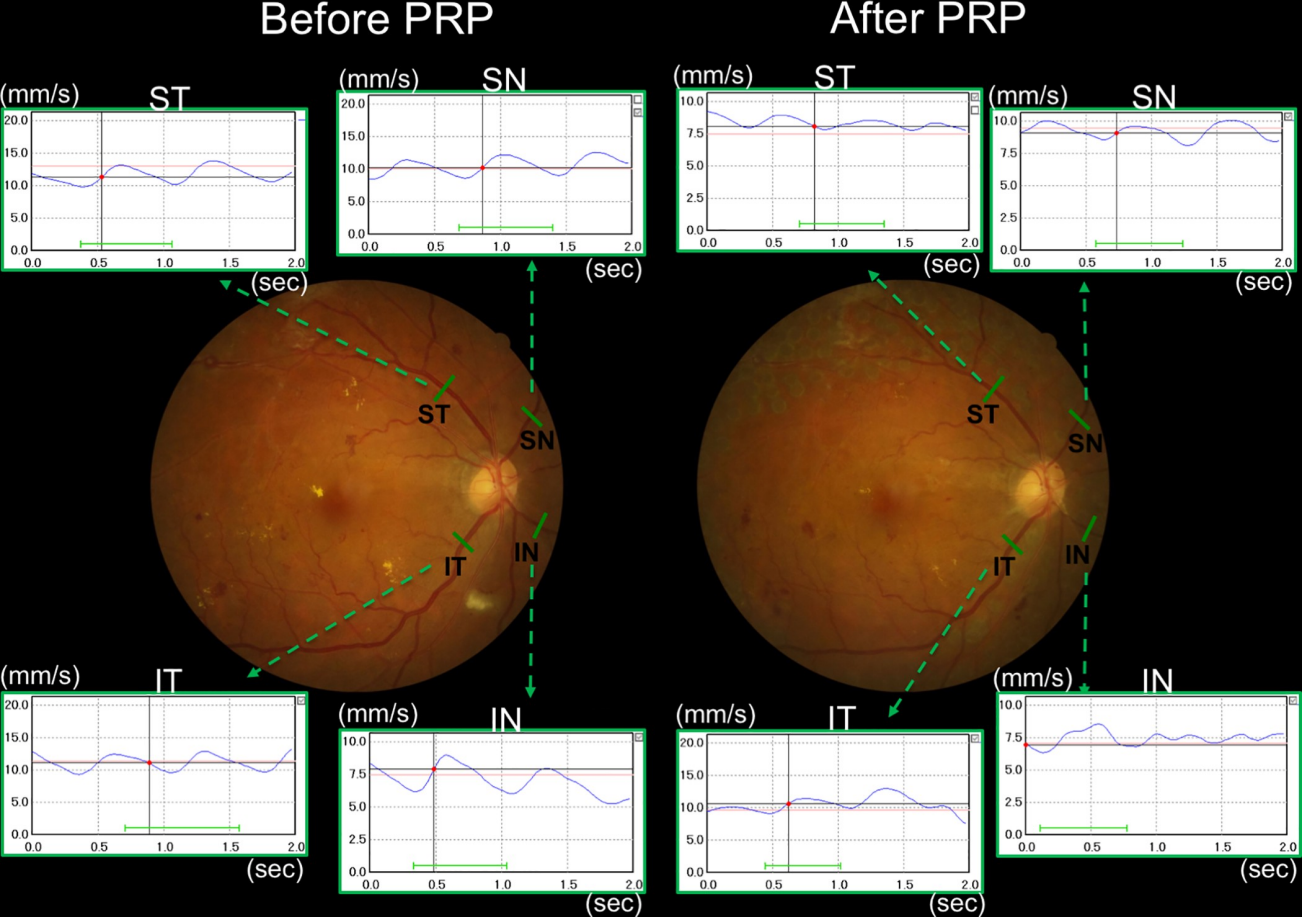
Examples of the site of venous measurements before (baseline) and after panretinal photocoagulation (Post PRP) and the waveforms of the blood velocity for the veins in before and after PRP eyes. The green bars indicate the inferotemporal (IT) vein, superotemporal (ST) vein, inferonasal (IN) vein, and superonasal (SN) vein. Each waveform of the veins were acquired by Doppler OCT flowmeter. The vertical lines indicate the retinal blood velocity (mm/sec) and the horizontal lines indicate time (sec). Before PRP, before starting PRP; After PRP, four weeks after completing PRP.
